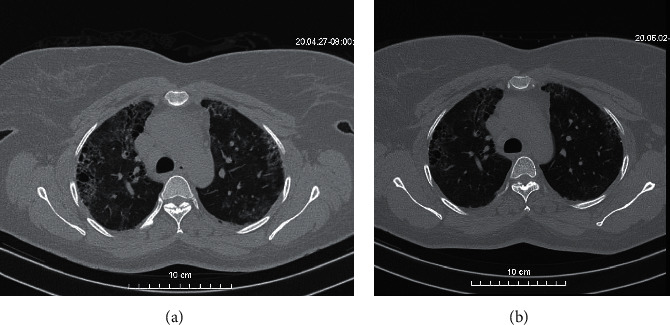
(a) Chest computed tomography (CT) scans (transverse plane) of the patient 1: frosted glass area extended central and bilateral upper lobar peripheral and fibrosis. (b) Chest computerized tomography (CT) scans (transverse plane) control of the patient 1: good evolution with almost disappearance of the frosted glass areas.
